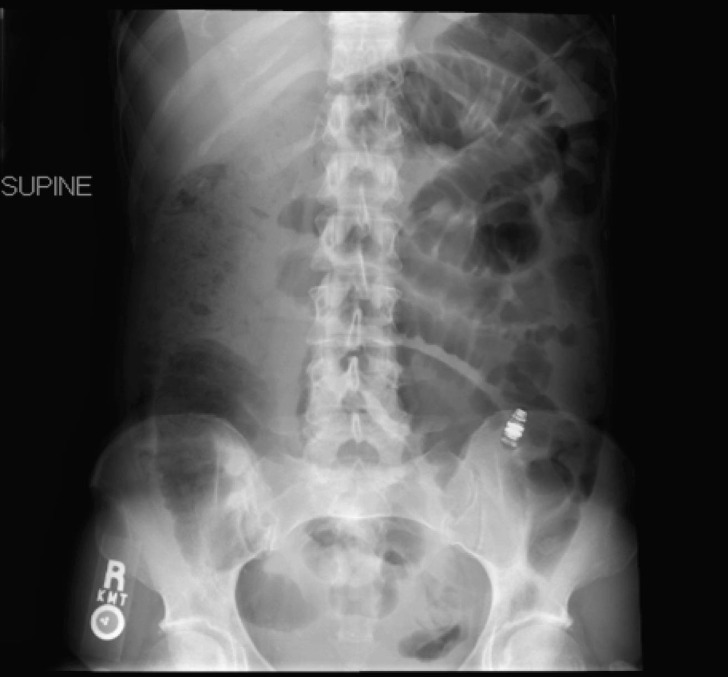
Abdominal radiograph showing a partial small bowel obstruction with retained capsule.
